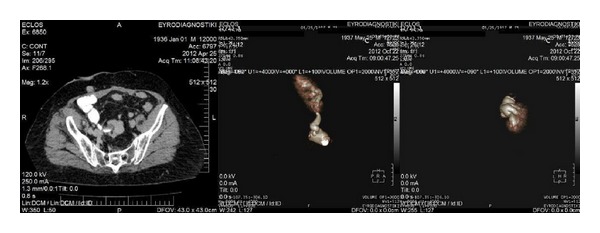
Postoperative and CT-pyelography (3D) of case 2.
